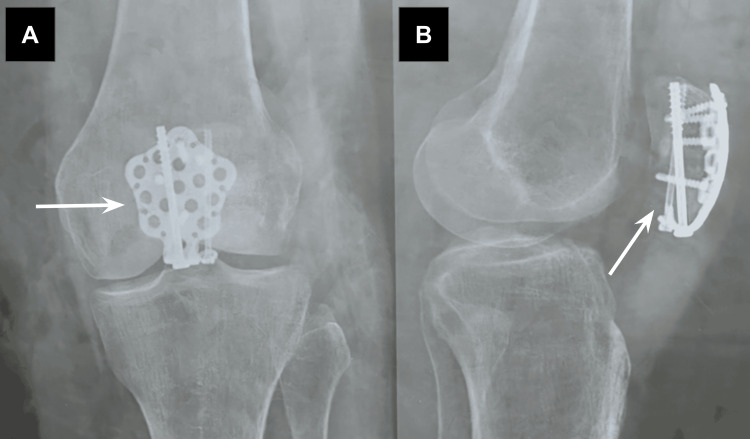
Left knee—anteroposterior (A) and lateral radiographs (B) indicating the result of the open reduction internal fixation procedure. Radiographs were obtained on postoperative day 1.
